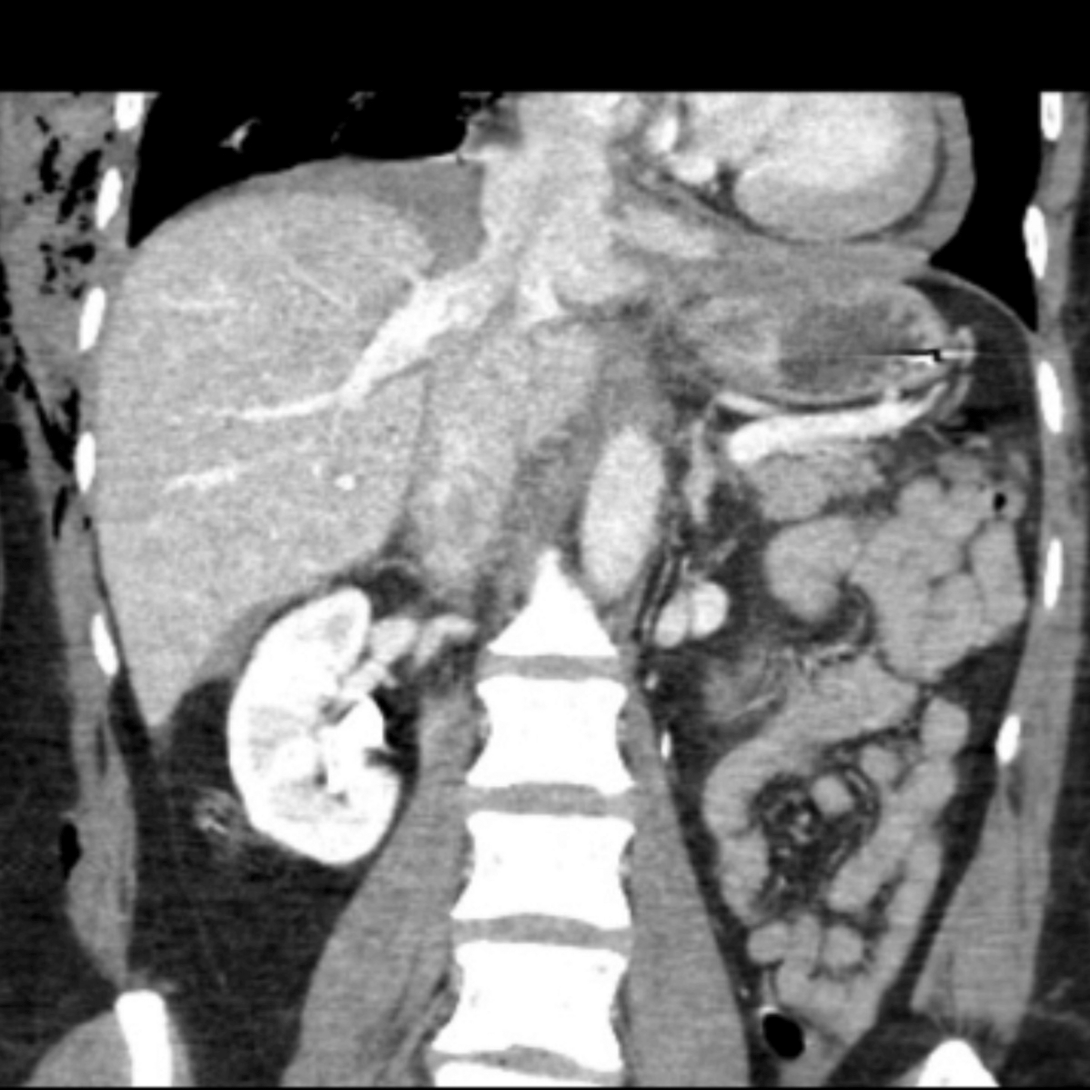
Radiological findings Coronal view of CT demonstrating IVC injury with active contrast extravasation and expanding hematoma within the diaphragmatic muscle CT: computed tomography; IVC: inferior vena cava
